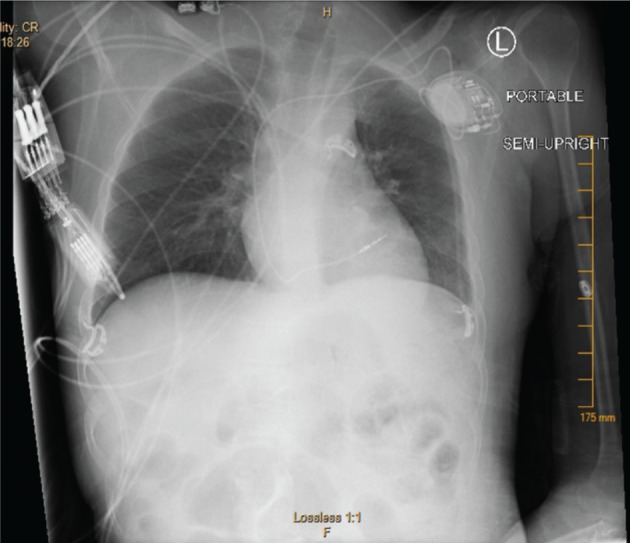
Chest radiograph revealing the patient’s single-chamber pacemaker with the ventricular lead placed in the septum.
